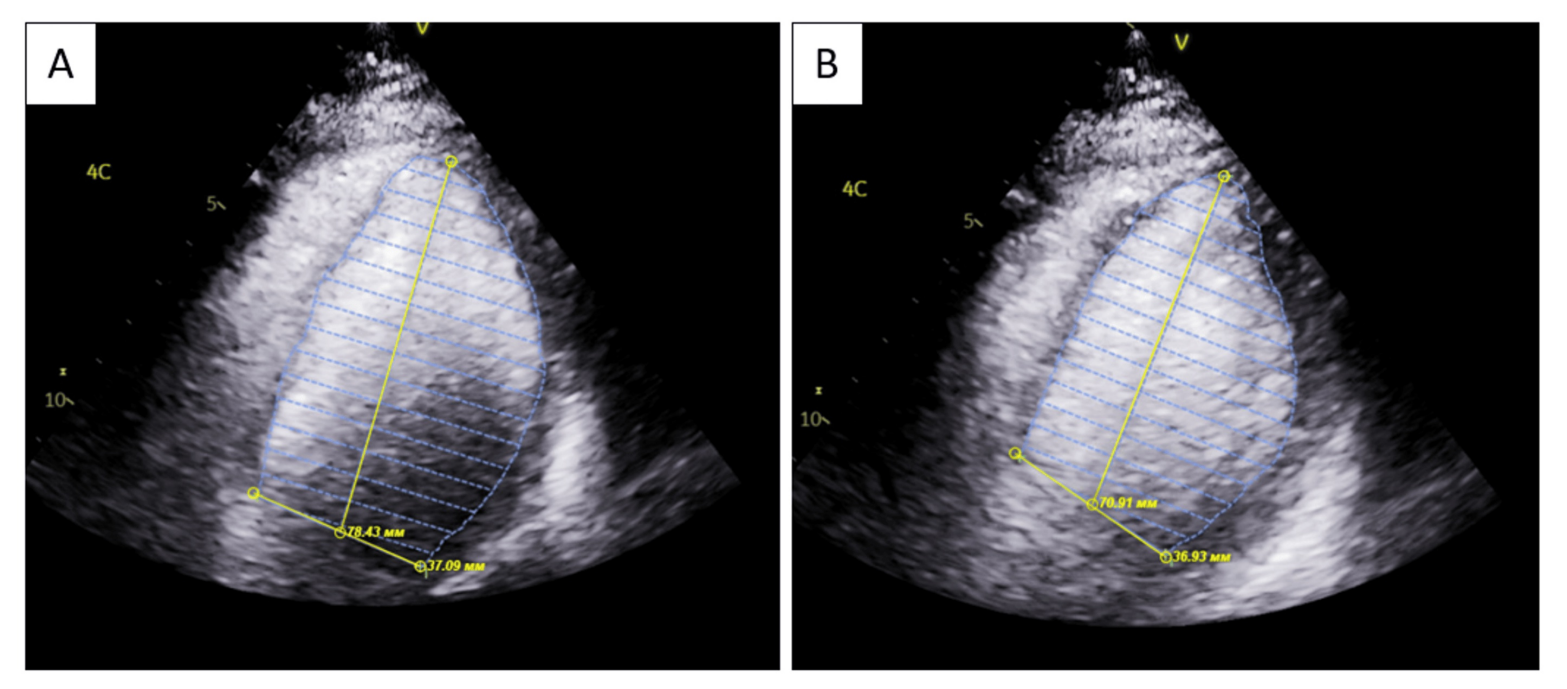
Transthoracic echocardiogram demonstrating reduced left ventricular systolic function. Apical four-chamber view. Panel A shows the left ventricle in end-diastole with increased left ventricular internal diameter in diastole (7.8 cm) and left ventricular end-diastolic volume measured in the apical four-chamber view (105 mL). Panel B shows the end-systolic phase with increased left ventricular internal diameter in systole (7.1 cm) and left ventricular end-systolic volume (85 mL), consistent with reduced systolic function. Linear dimensions and volumetric measurements were derived using different echocardiographic methods, which may account for the apparent discrepancy between diameters and calculated volumes.
